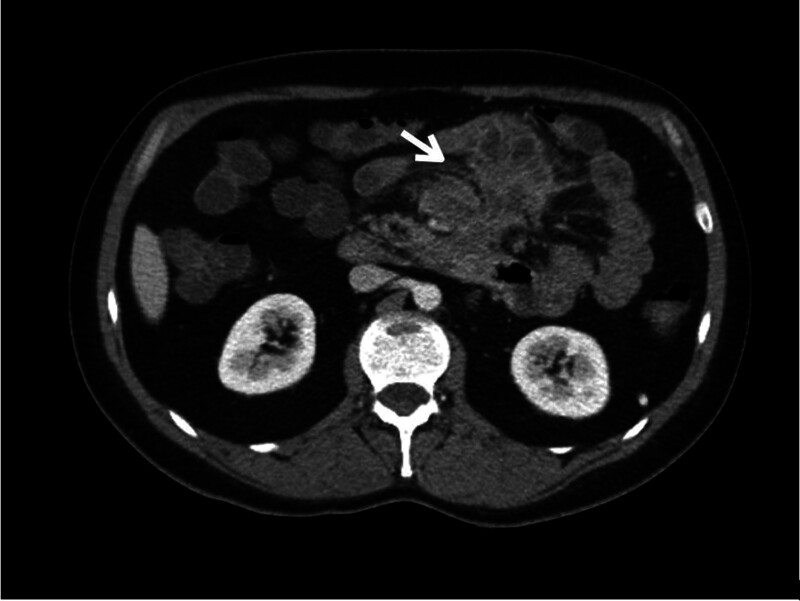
Images of the CT-scan performed in May 2021. White arrow shows mesenteric sclerosis.
